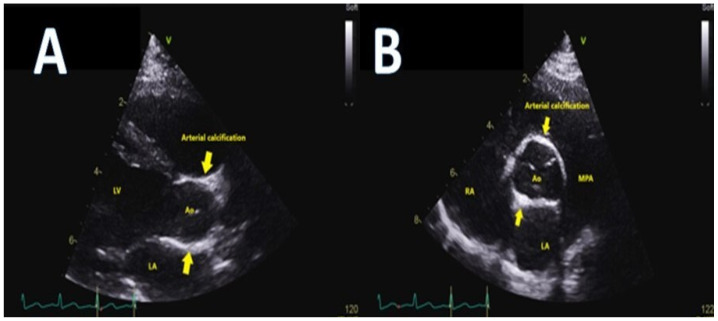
Thickening of the aorta patient seen in echocardiographic parasternal long axis (panel (A)) and short axis (panel (B)) views in arterial calcification syndrome.
